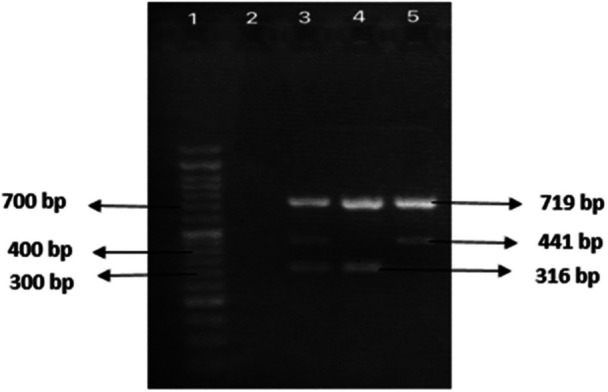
The electrophoresis results obtained from the T‐Arms‐PCR amplification of SFTPD gene SNPs (rs721917, T > C) have been visually shown on a 2% agarose gel. Lane 1: 50‐bp ladder (Cinnagen, Iran); Lane 2: no template control; Lane 3: CT heterozygote; TT homozygous mutant; and Lane 5: CC homozygous reference genotype.
